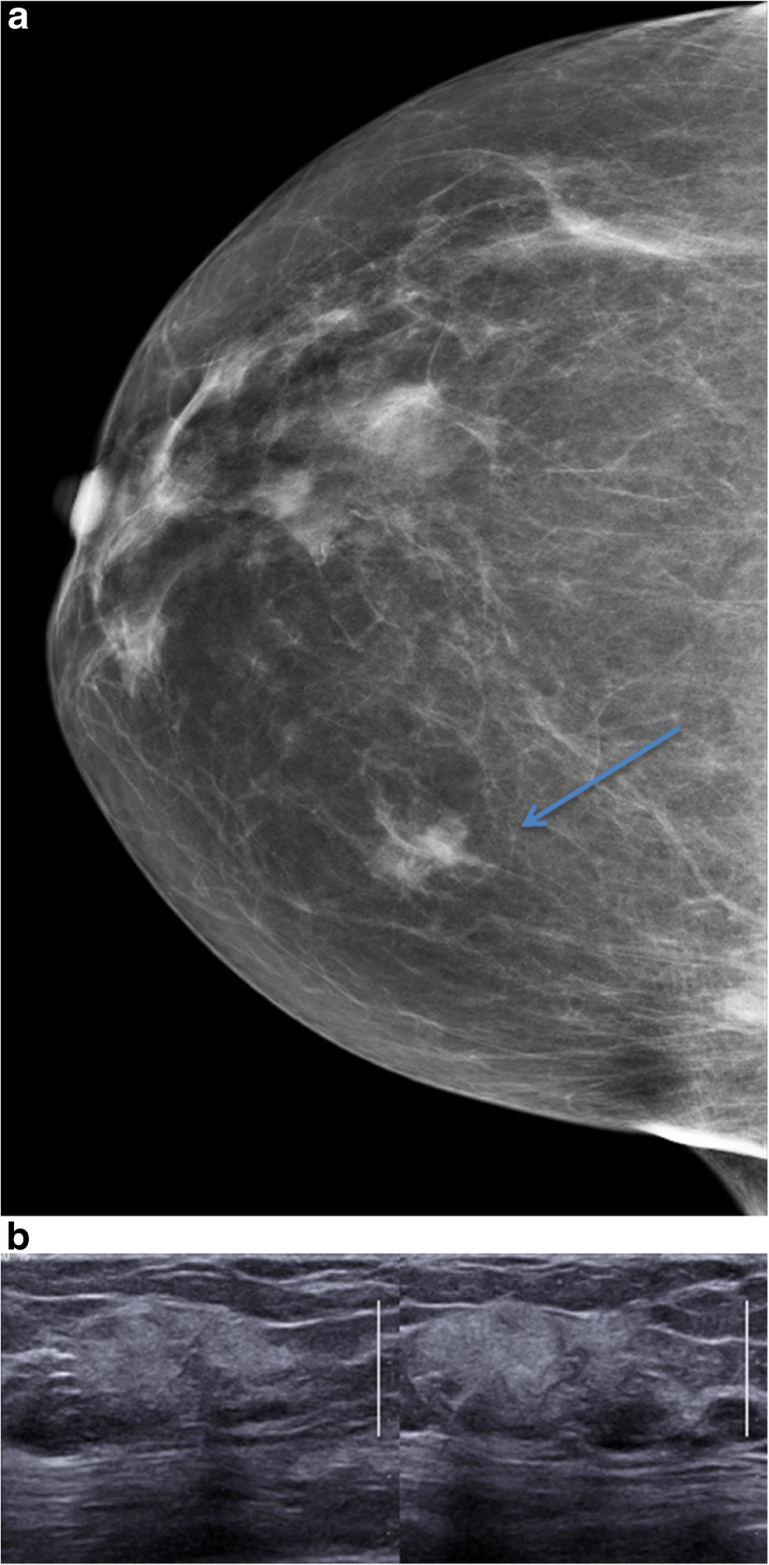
Invasive lobular carcinoma. a Mammography, AP view, showing a mass with irregular margins in the right upper inner quadrant (*arrows*). b Ultrasound showing an irregular, poorly circumscribed, vertically oriented, hyperechoic and partially attenuating lesion
